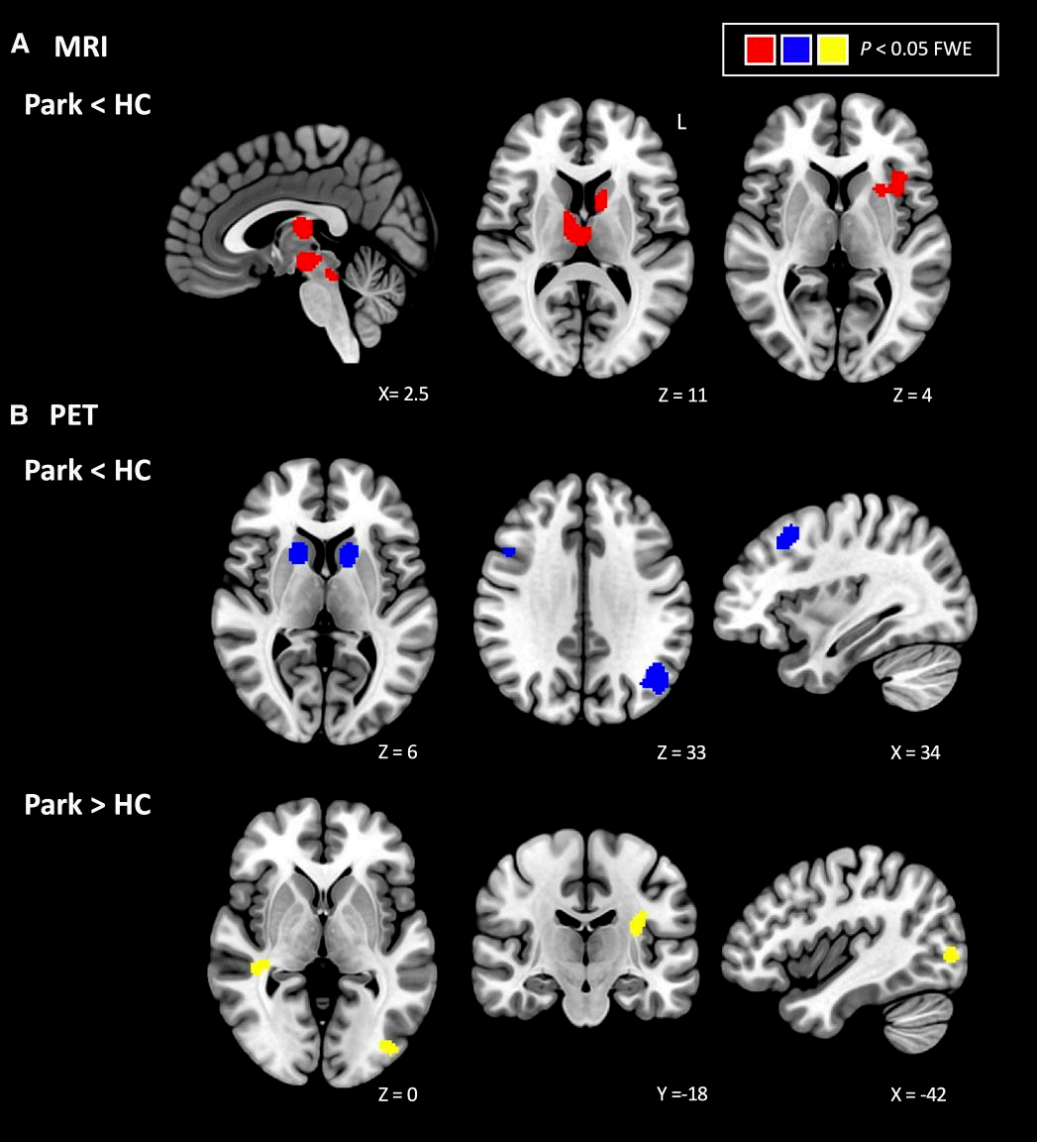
Meta-analysis findings across parkinsonian disorders. (A) Meta-analysis of MRI imaging in all parkinsonian disorders, patients < HC (top row). (B) Meta-analyses of PET imaging in all parkinsonian disorders, patients < HC (middle row) and patients > HC (bottom row). FWE = family-wise error; HC = healthy controls; Park = parkinsonian disorders.
